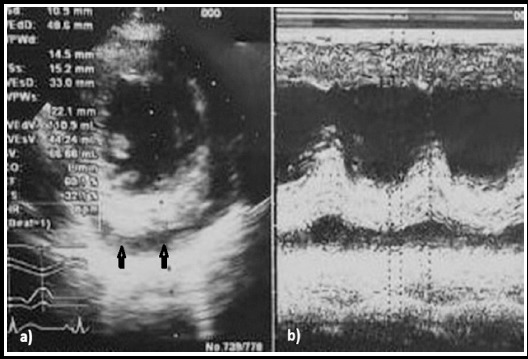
Echocardiography images a) parasternal short axis view (PSS) and b) M mode shows mild pericardial effusion (arrows) due to infective pericarditis in 32 years old patient of Tuberculous infective pericarditis.
